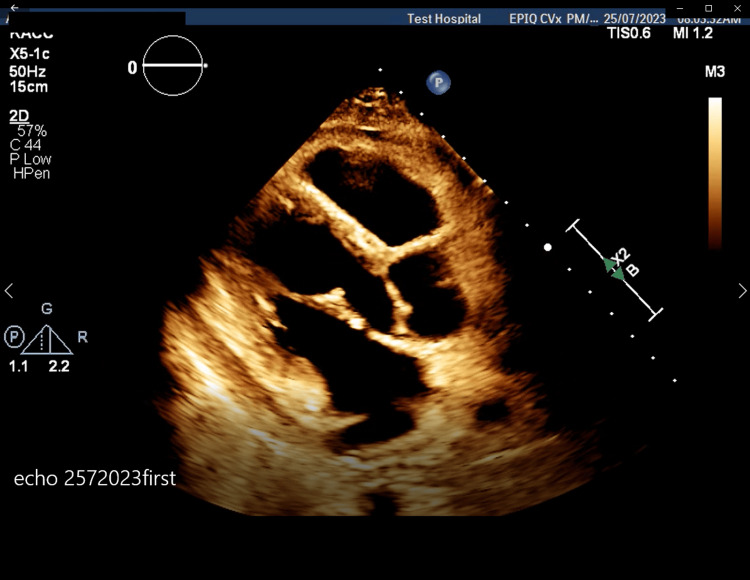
Echocardiography performed on 25/07/2023 revealed right ventricular dilation with mild to moderately reduced systolic function (RV free wall global longitudinal strain (RVFW-GLS): -15.9%), pericardial thickening, and a small pericardial effusion.
